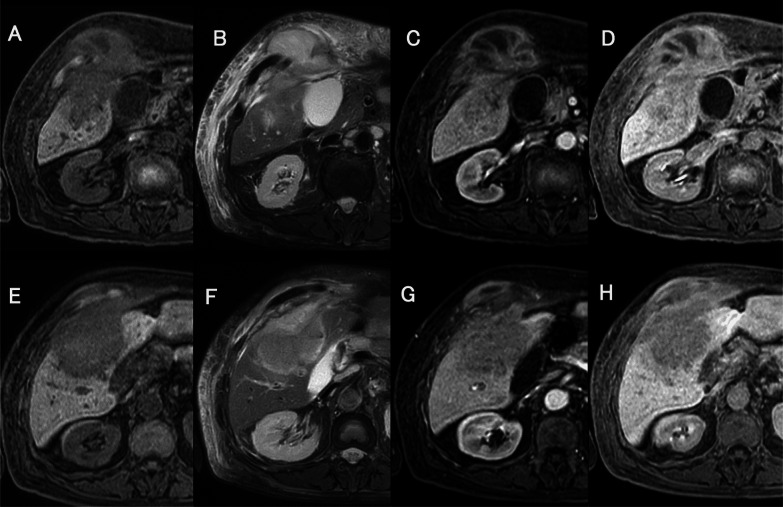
Enhanced magnetic resonance imaging before admission (A, E T1-weighted image, B, F T2-weighted image, C, G arterial phase, D, H hepatobiliary phase; A–D cranial site of the mass, and E–H caudal site of the mass)
